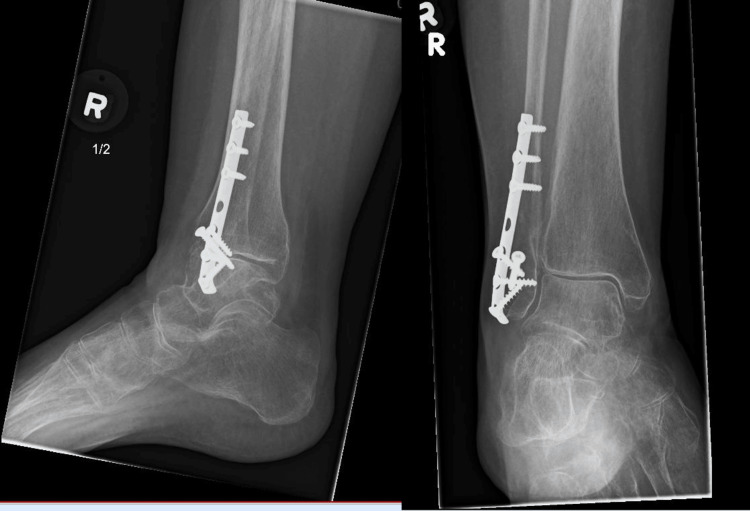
Postoperative X-ray of Weber B fracture.
